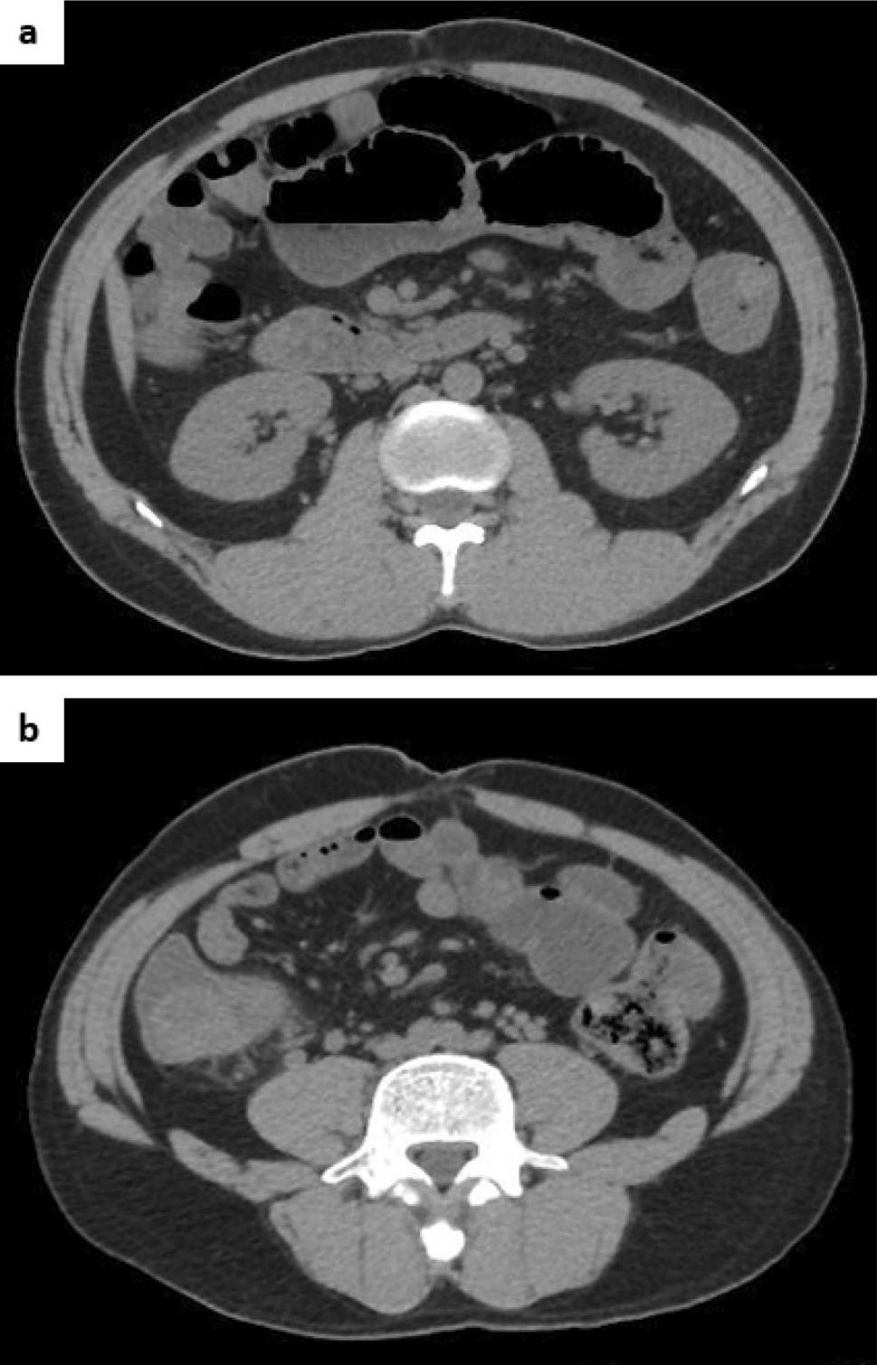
Abdominal plain computed tomography performed in December 2017. a Discontinuous dilation in the small and large intestine. b Wall thickening of the ascending colon from the cecum near the ileocecal region
